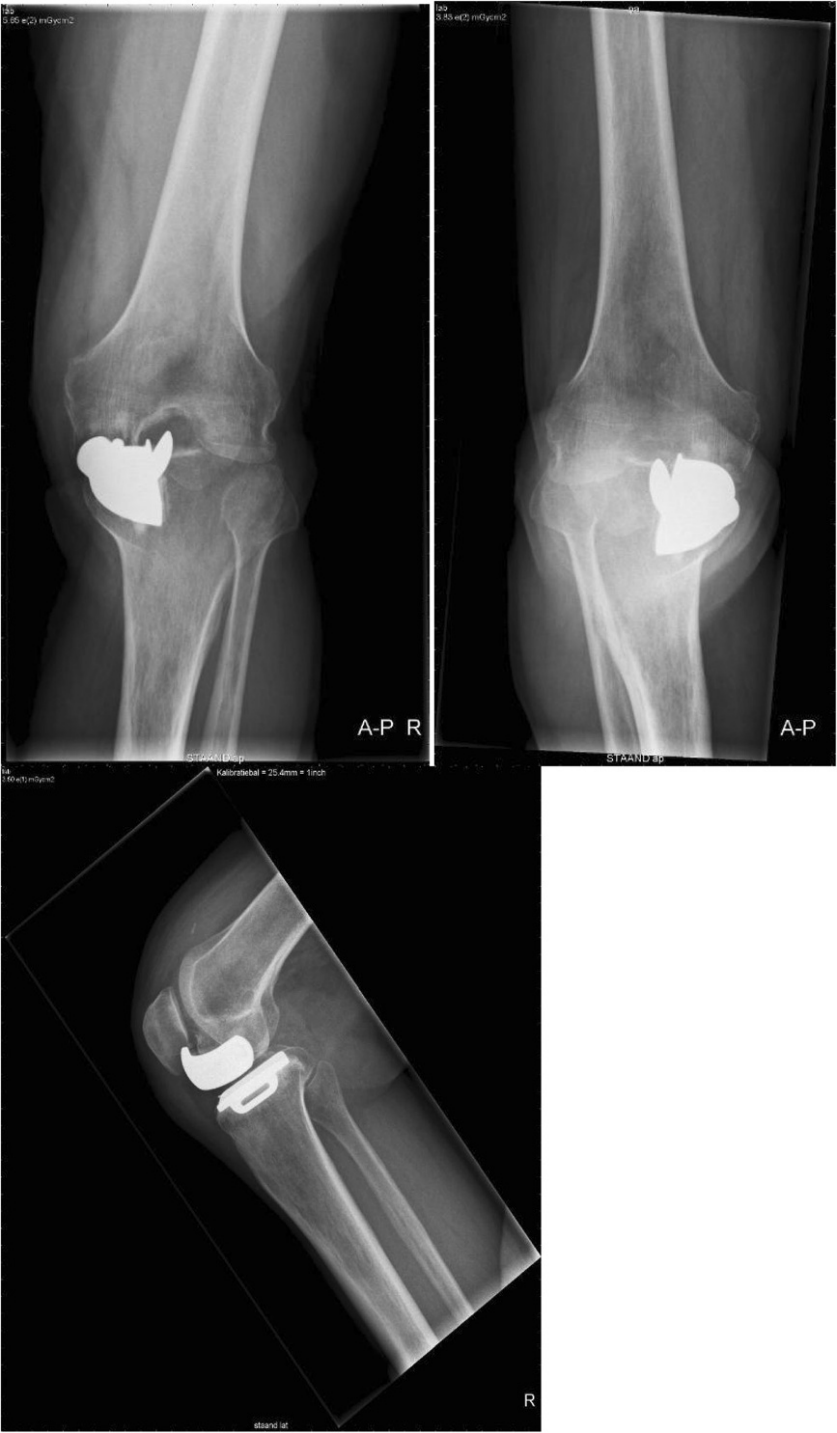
Anterior-posterior and lateral X-rays at 2 weeks after the 3-month postoperative check-up with the patient reporting extreme pain and a locked knee.
